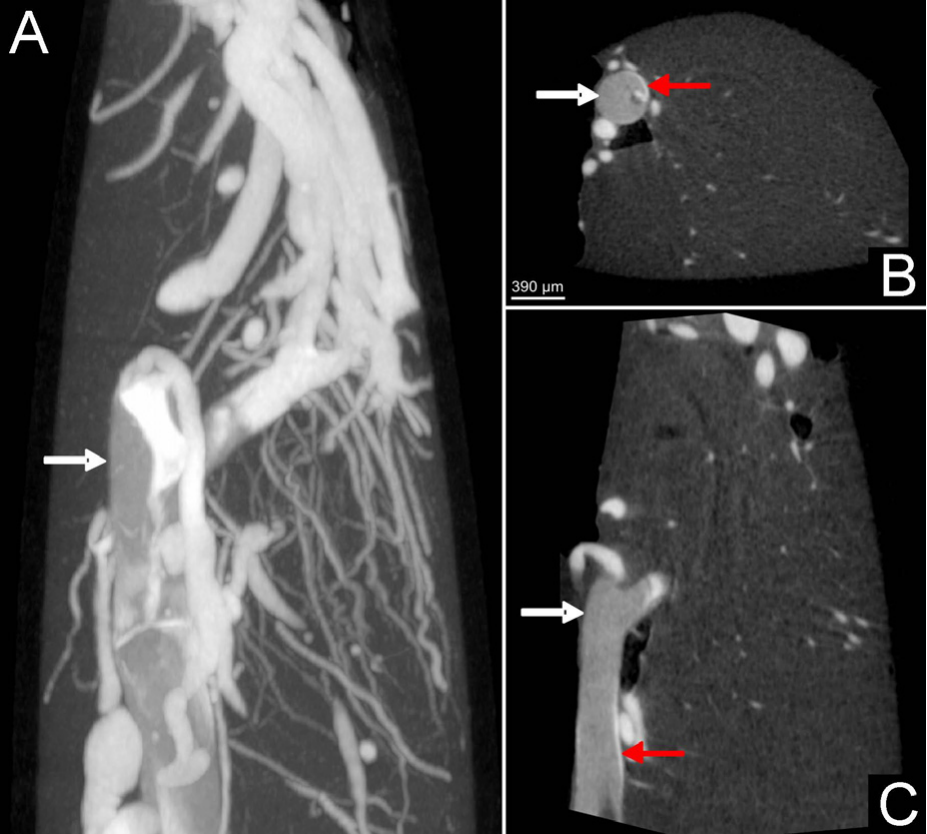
Contrast agent at the top of the suture (white arrow) indicating partial perfusion in the anterior cerebral artery as demonstrated with nano-CT imaging. Note the sparse contrast perfusion between the suture and the vascular wall (red arrow) indicating the non-complete occlusion of the vessel.
